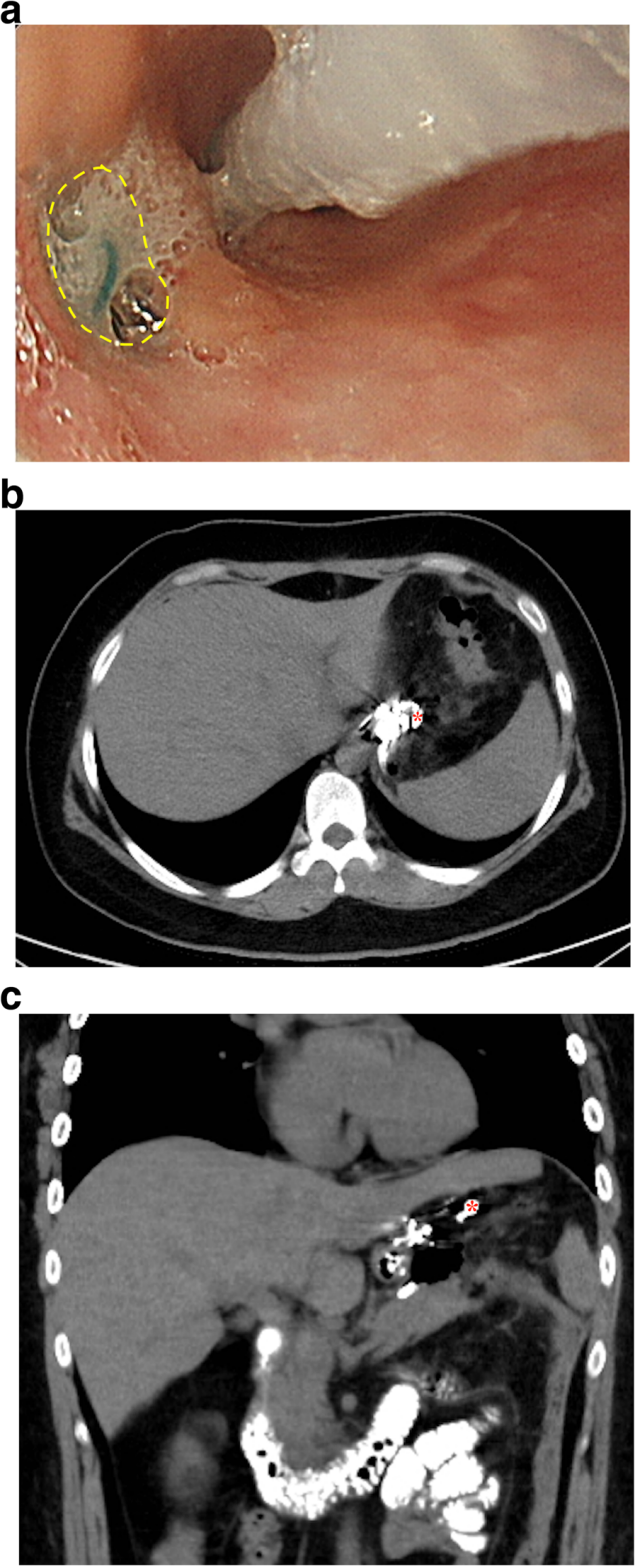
Endoscopic glue injection for closure of the fistula at POD40 (a), and an oral contrast enhanced CT scan one week, at POD48, after endoscopic glue injection (b and c). An about 5 mm fistula tract opening was found via endoscopic examination (dashed line, a), and was closed by Histoacryl® and Lipiodol® injection. The following oral contrast enhanced CT scan one week later still showed a minor leak (asterisk, b and c) and a more enlarged size of the pseudocavity. Abbreviation: POD, postoperative day; CT, computed tomography
